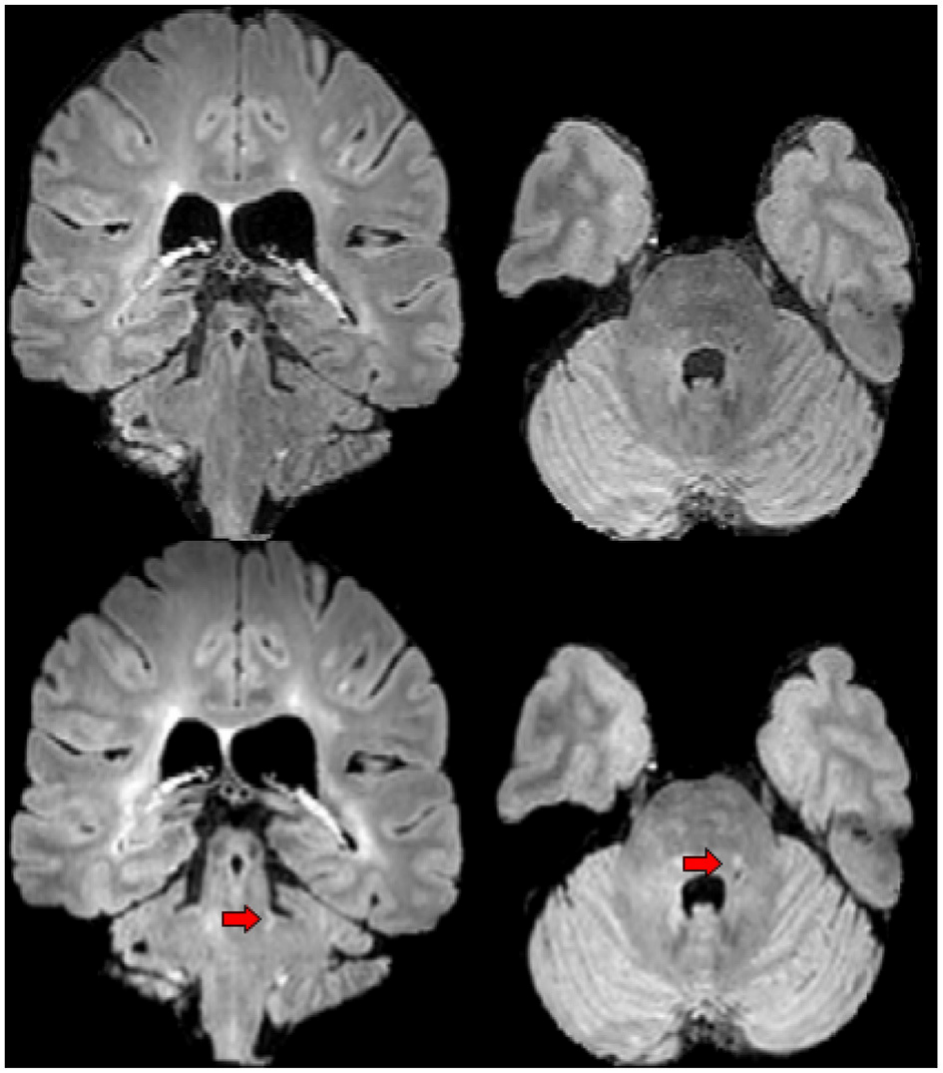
An example of lesion detected by expert 1 in the first phase of the experiment but not by experts 2 and 3. First row shows the baseline FLAIR scan (from left-to-right: coronal and axial view), second row shows the FLAIR scan 1 year later (from left-to-right: coronal and axial view). Red arrows designate the lesion of interest.
